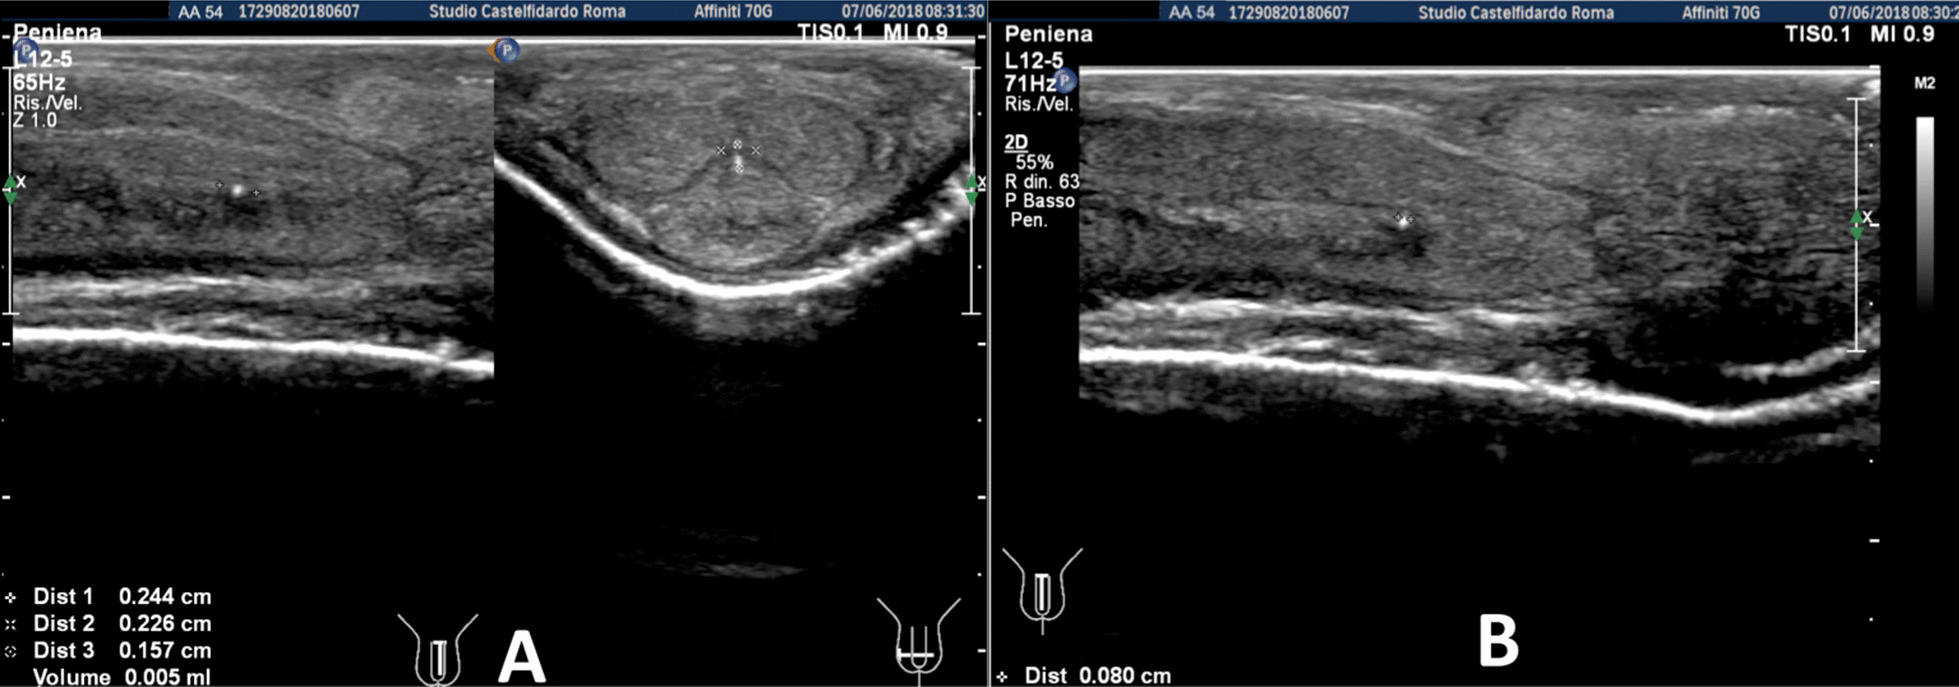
Penile ultrasound after the fourth treatment cycle (12 months). A Plaque measurement in longitudinal and transverse scan. B Calcification measurement in longitudinal scan
